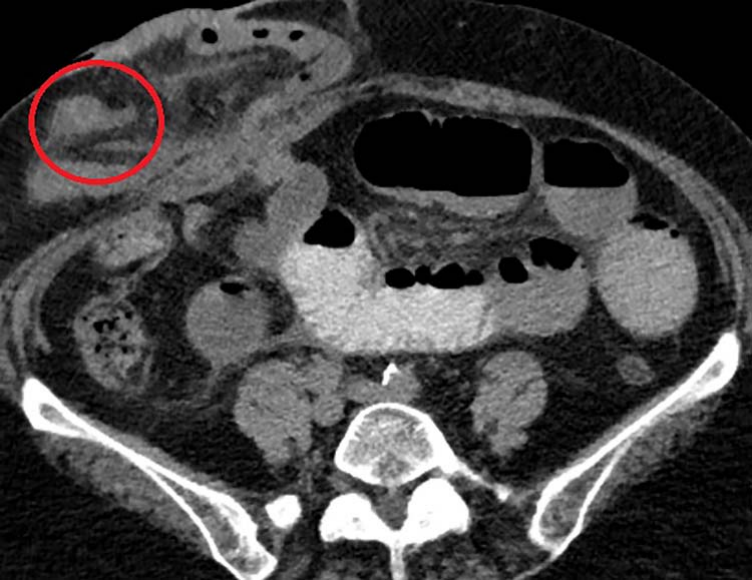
Axial CT scan demonstrating the MD (circled) arising from the anti-mesenteric aspect of the ileum within the parastomal hernia, identified after close retrospective review of imaging post operatively.
